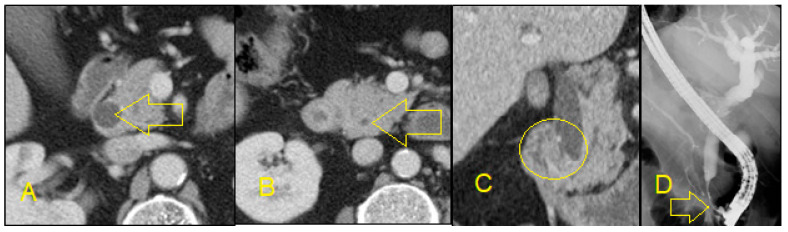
A: Axial contrast-enhanced CT shows enhancing dilated common duct (arrow); B: there is abrupt cutoff of the common duct and there is diffuse mural thickening and enhancement (arrow); C: The circle demonstrates an infiltrative mass in the distal duct found on coronal CT; D: endoscopic retrograde cholangiopancreatography (ERCP) shows a short-segment tight stricture corresponding to the mass. Biopsy confirmed cholangiocarcinoma.
